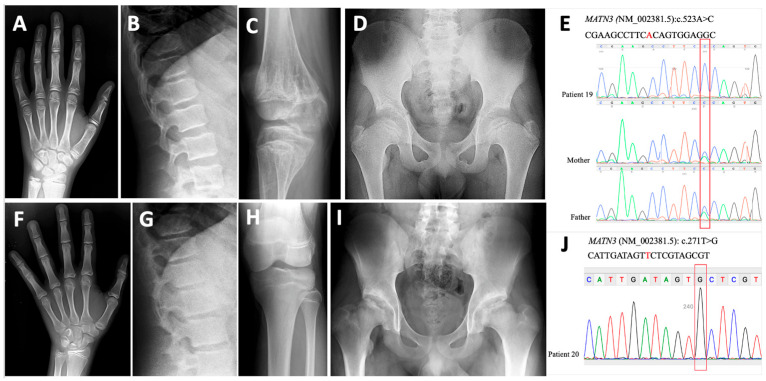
Patients with MED5. P19 at the age of 14 years. Note mild carpal irregularity (A) and platyspondyly (B), severe irregularities in the knee epiphyses with metaphyseal striae (C), flattening of the femoral head, and coxa vara (D). Sanger sequencing of the VUS identified in P19 and segregation in the family (E). Radiographs of P20 with MED5 at the age of 14 years showed mild carpal irregularity (F) and platyspondyly (G), a normal knee joint (H), and acetabular irregularity with destroyed femoral heads (I). Sanger sequencing of the VUS identified in P20 by exome sequencing (J).
